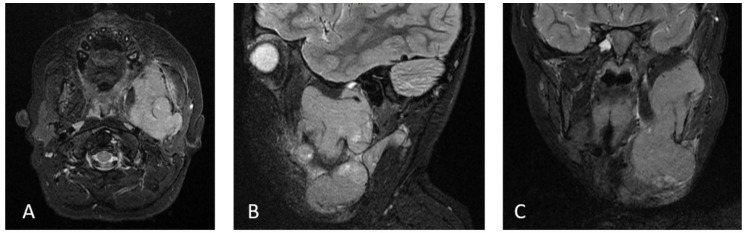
MRI of the face and mandible with and without contrast at the diagnosis of secondary PNET. The images show Short-TI Inversion Recovery (STIR). They demonstrate the mandibular mass. (A) Axial, (B) Sagittal, (C) Coronal.
